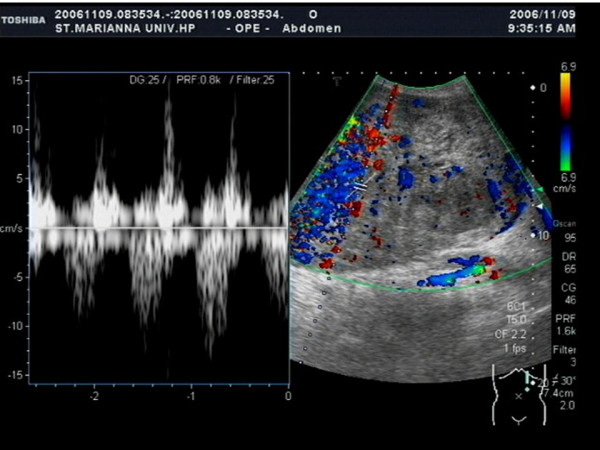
Doppler ultrasonography shows hypervascular area in the tumor.
